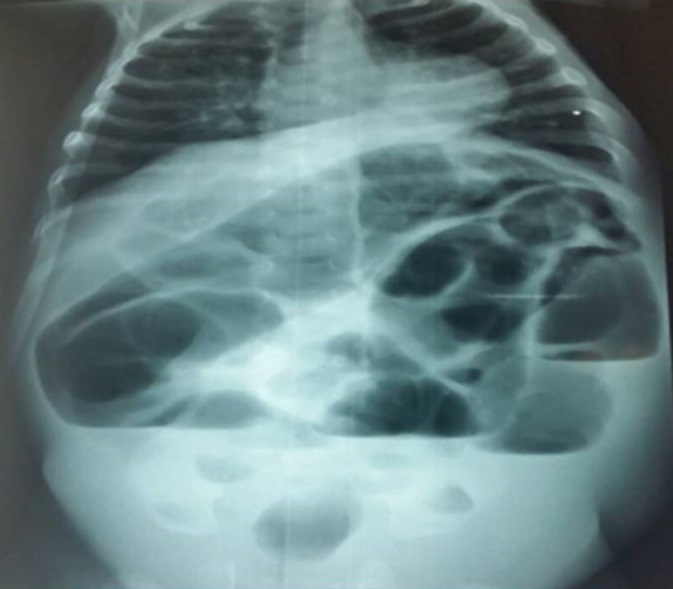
Une radiographie thoraco-abdominal post natale objectivant une distention grélique
